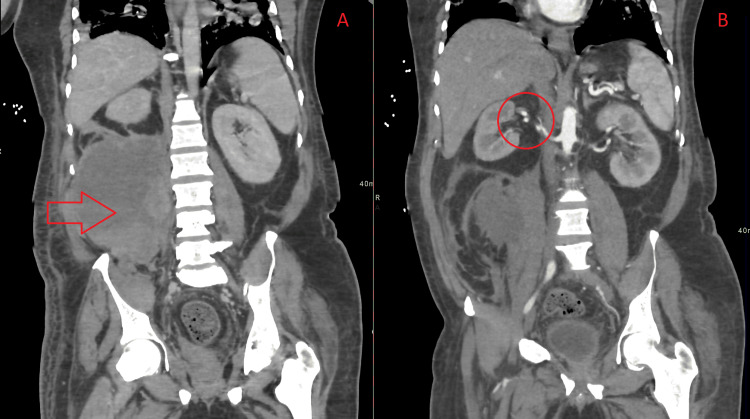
CT of the abdomen and pelvis. A) Right retroperitoneal hematoma (red arrow). B) Right renal artery non-occlusive filling defect (circle).
